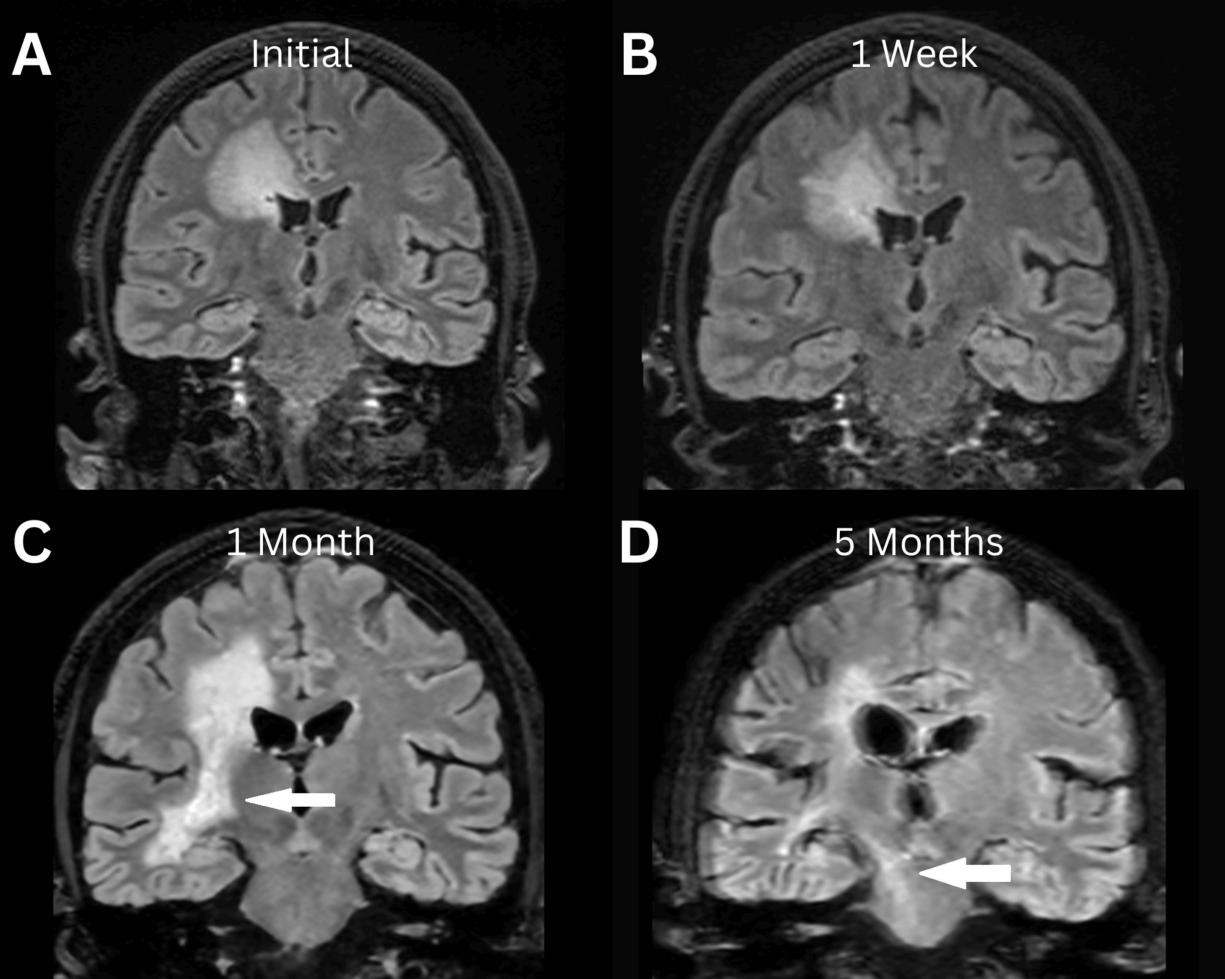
Spread of T2 FLAIR signal to the brain stem On coronal views, the T2 fluid-attenuated inversion recovery (FLAIR) signal can be seen extending from the periventricular white matter and descending toward the brain stem at different time points from the initial presentation. (A) One week after presentation, (B) One month after presentation, and (C) Five months after presentation. (D) White arrows in images C and D show the new areas of involvement over time.
